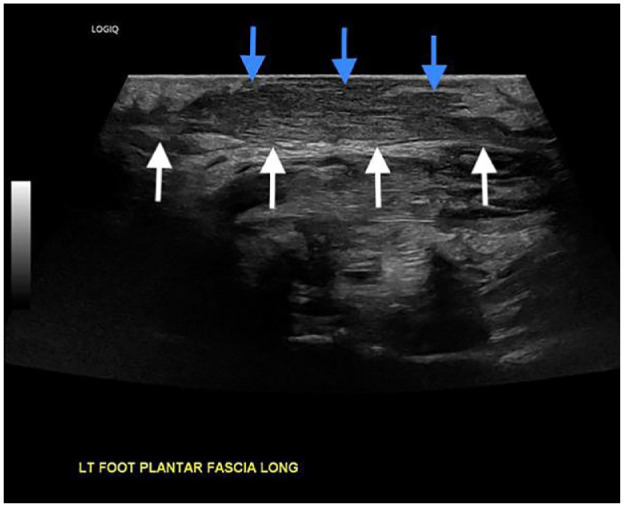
Six-month follow-up static longitudinal sonographic image of the plantar fascia (white arrows) at the location of the prior mass, demonstrating near complete resolution of the plantar mass with residual superficial postsurgical scarring (blue arrows) present.
